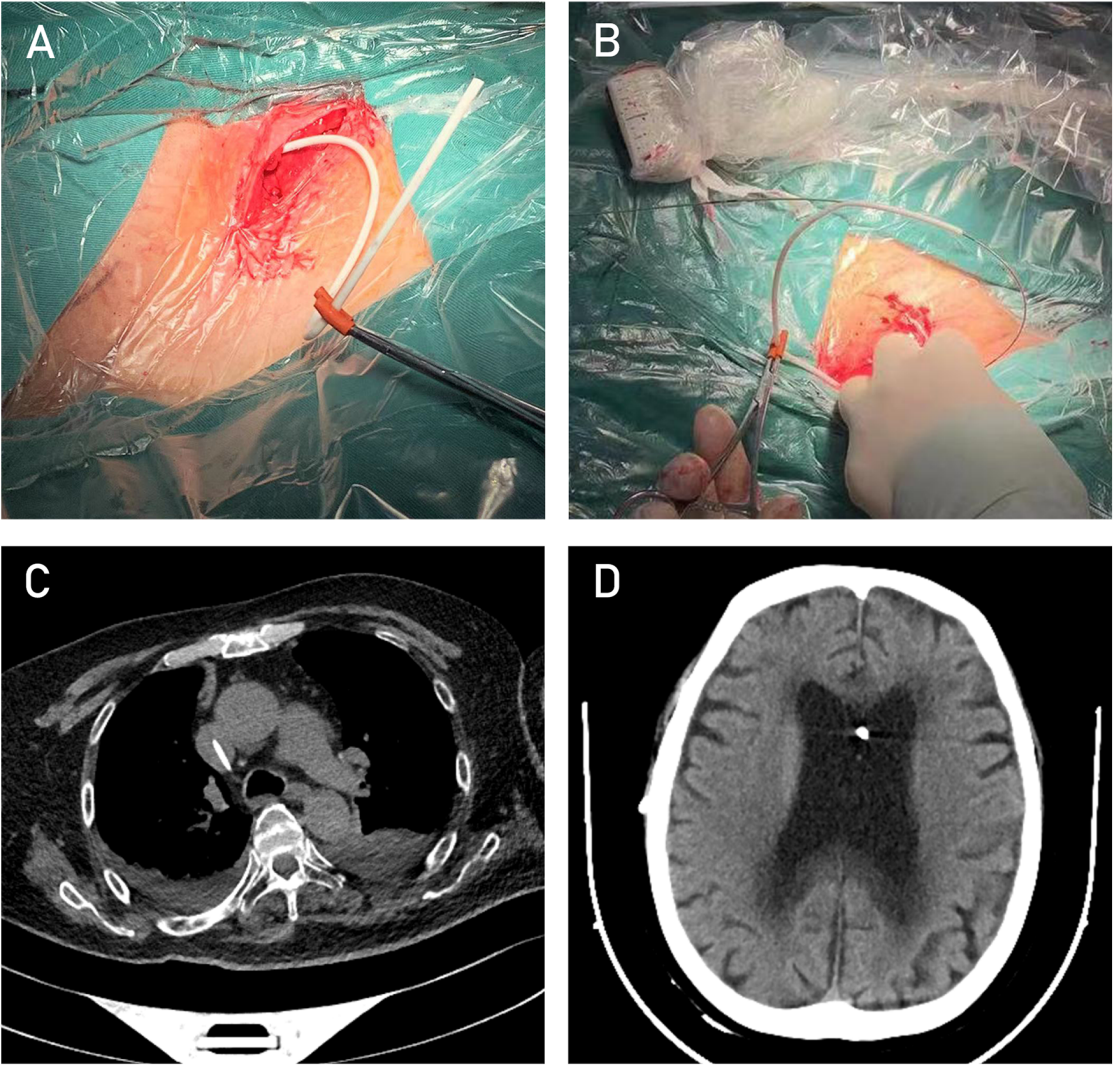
Intraoperative image after completion of Step 1. (A) Intraoperative image after completion of Step 2. (B) Post-VA shunt cardiac CT image showing the catheter in the target position. (C) Post-VA shunt follow-up CT image of the lateral ventricles (D).
